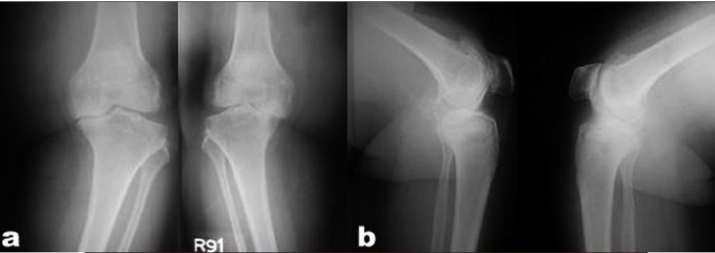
Bilateral anteroposterior (a) and lateral (b) knee radiographs of an obese patient with advanced knee osteoarthritis
